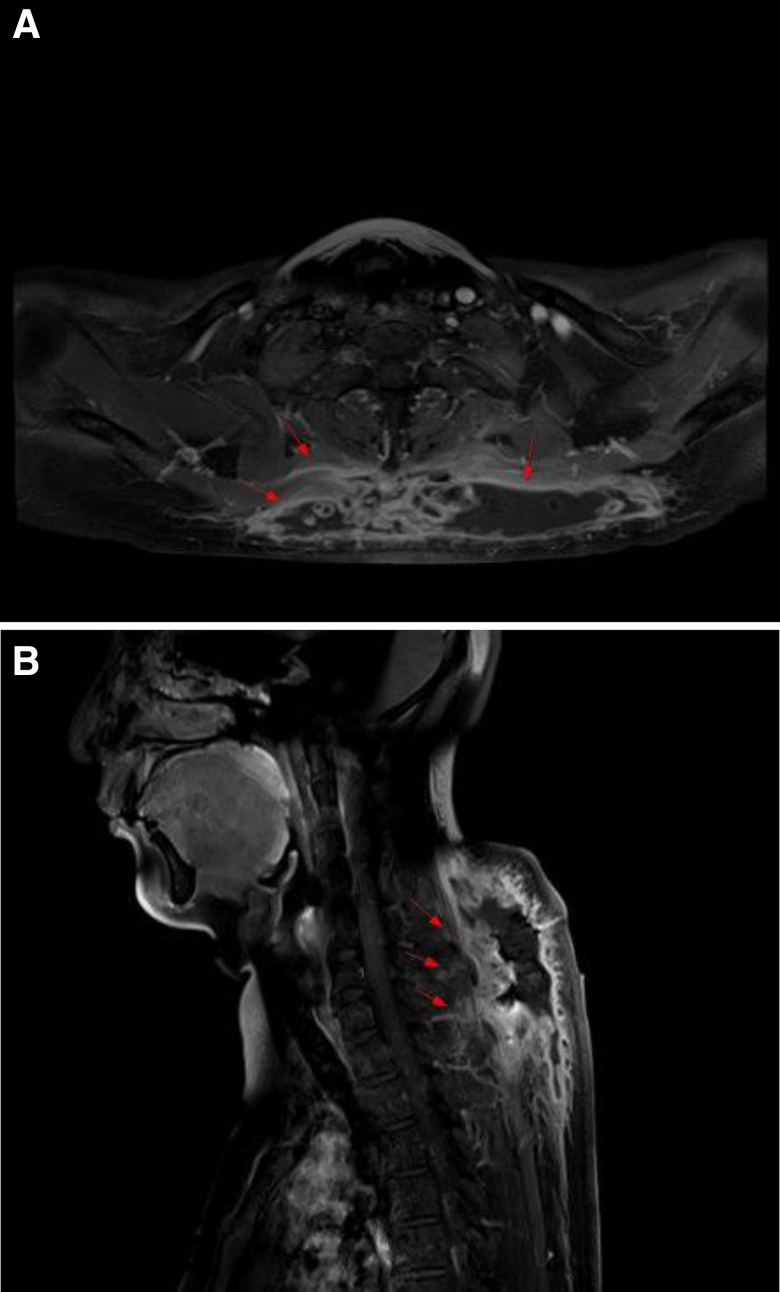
MRI following incision and drainage (upper back). MRI scan of the patient’s upper back after incision and drainage. (A) Skin and soft-tissue defect measuring 20 × 12 × 3.5 cm with necrotizing fasciitis of the left trapezius and levator scapulae. (B) Sagittal view showing superficial and deep fasciitis with involvement of the back muscles. MRI = magnetic resonance imaging.
